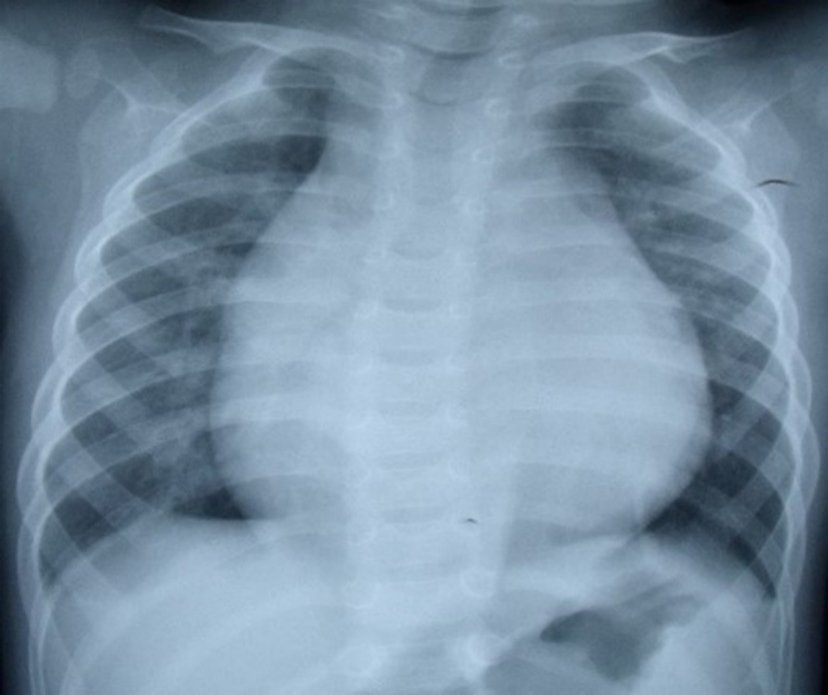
Chest X-ray:cardiac enlargement and cardiothoracic ratio 0.68.
